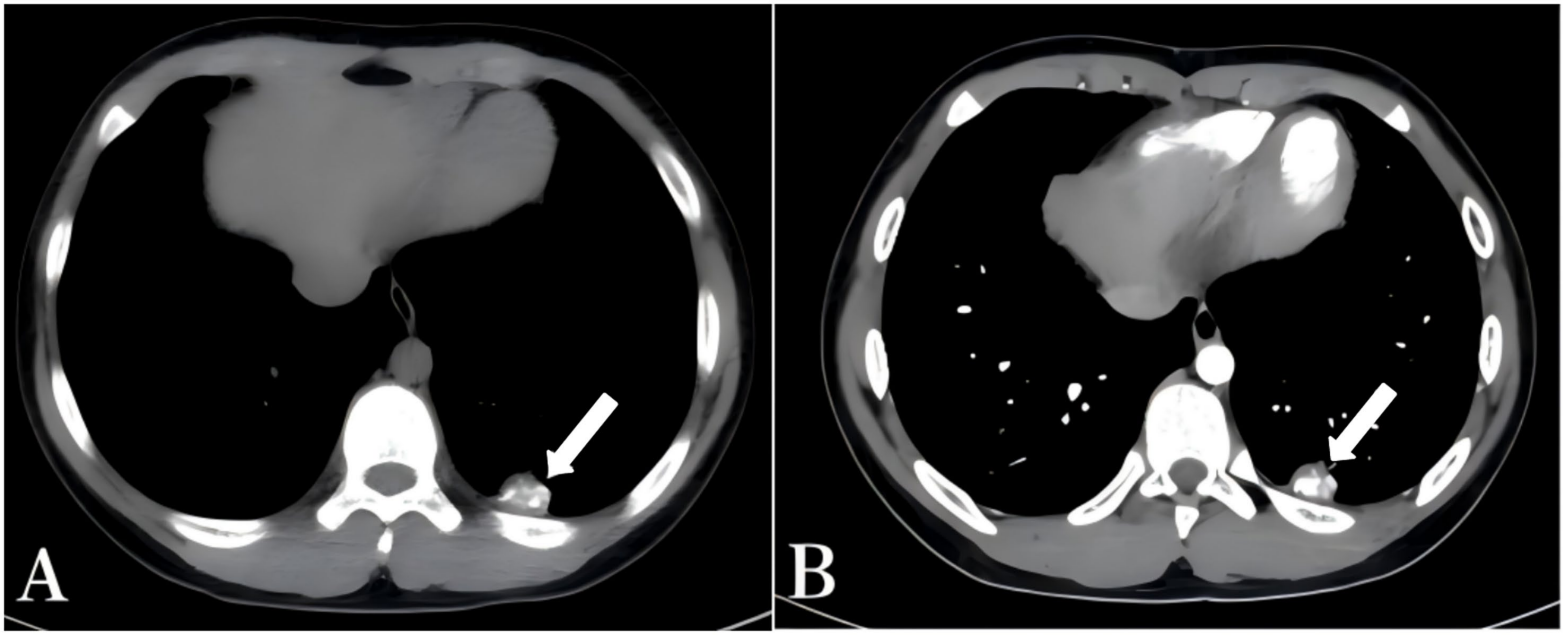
(A) Non-contrast CT reveals a mixed-density mass in the posterior basal segment of the lower lobe of the left lung, with a maximum cross-sectional dimension of approximately 23 mm × 18 mm. Multiple calcified foci are visible within the mass (arrow). (B) Contrast-enhanced CT shows heterogeneous enhancement of the lesion (arrow).
